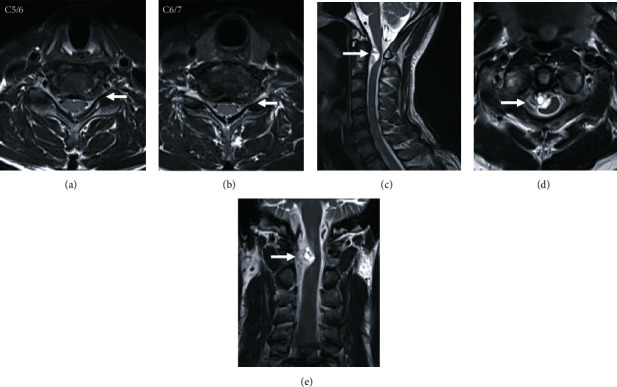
Noncontrast-enhanced magnetic resonance images. In horizontal sections (a, b), cervical disc herniation happened in the same region as the left C5-6 and C6-7 stenoses (arrow). At the C1-2 level, an intradural extramedullary cystic lesion existed in the right side of ventral cervical spinal cord, which severely compressed the spinal cord ((c) sagittal, (d) horizontal, and (e) coronal, arrow).
